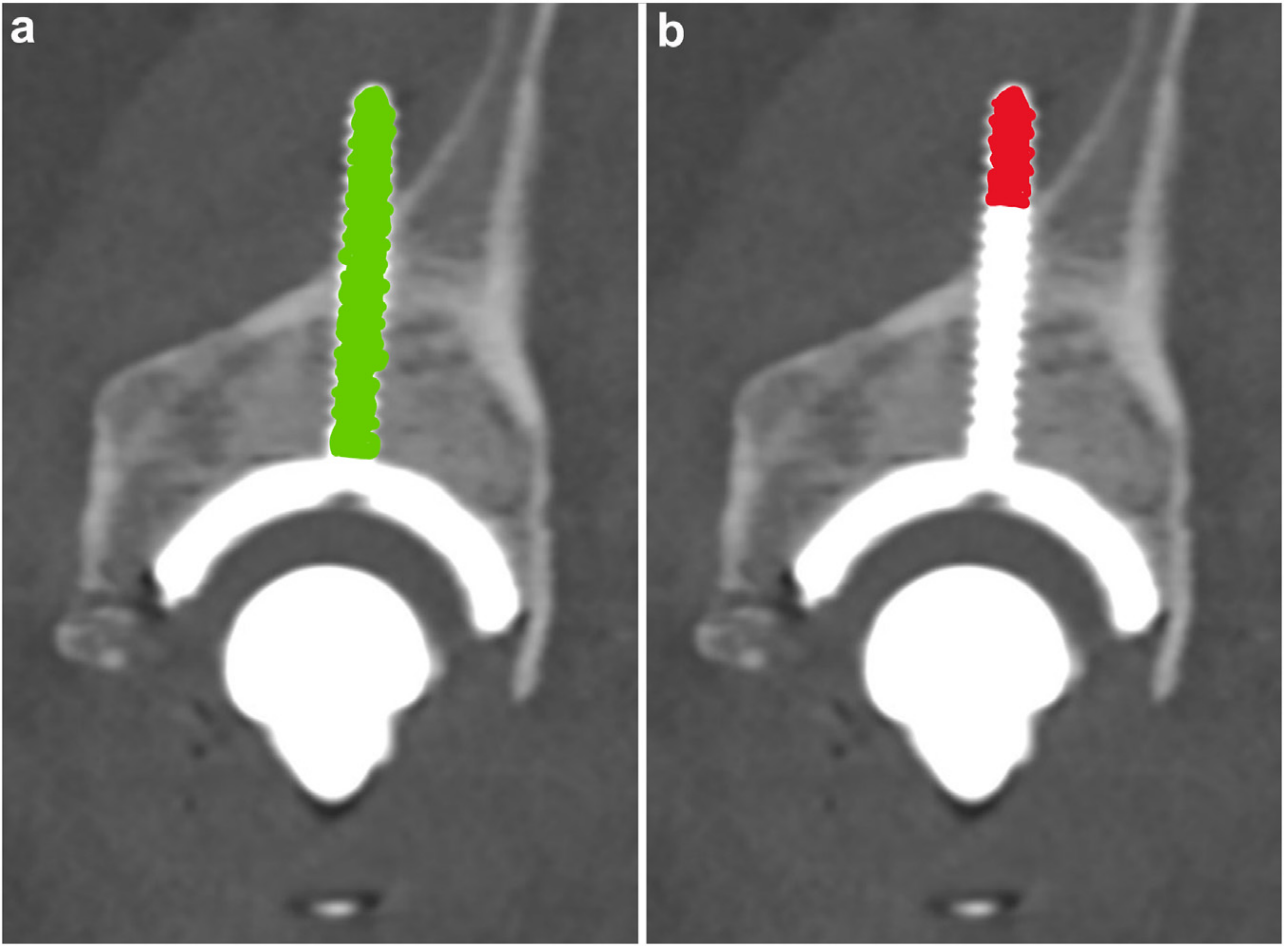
Measurement of screw length in the multiplanar reformation view of a postoperative CT image. Before the measurement of screw length, the 3 planes were aligned along the screw centerline. The *total screw length* (green/a), which is defined as the distance from the outer cup surface to the very tip of the screw, and *screw penetration depth* (red/b), which is defined as the distance from the last screw thread in contact with bone to the screw tip, were measured for each screw.
